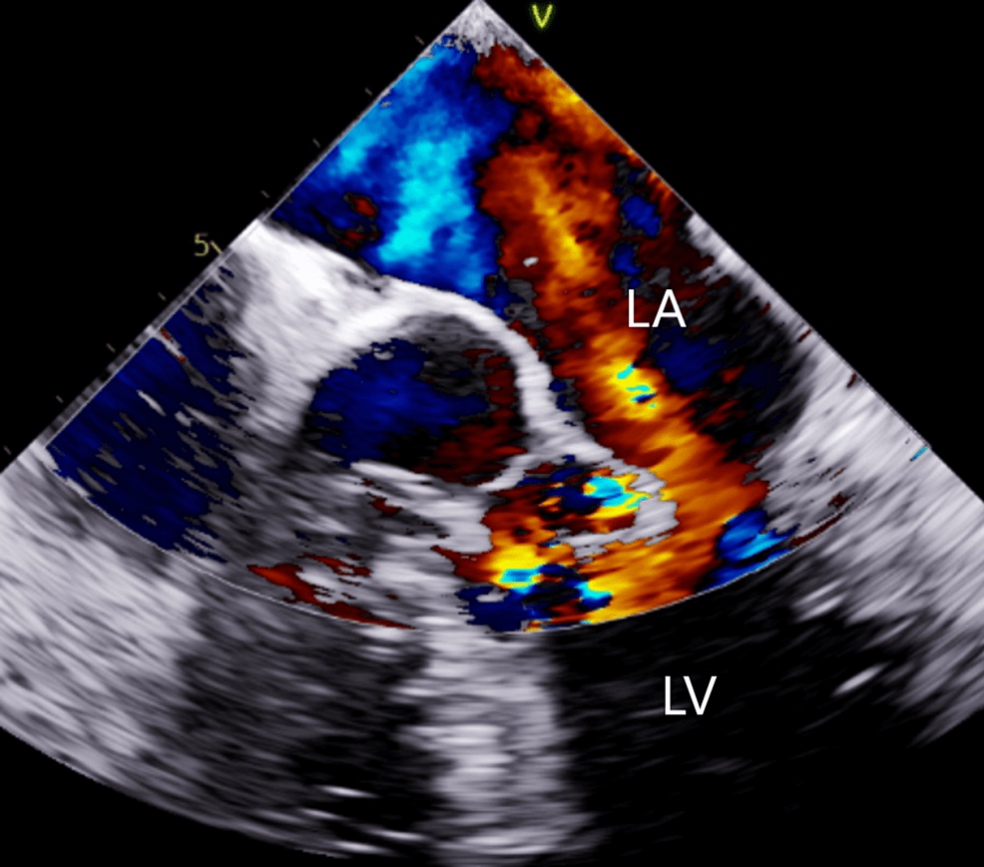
Transesophageal echocardiogram mid-esophageal 4-chamber view showing mitral valve prolapse with wide-open regurgitation (red area). LA: Left Atrium; LV: Left ventricle
